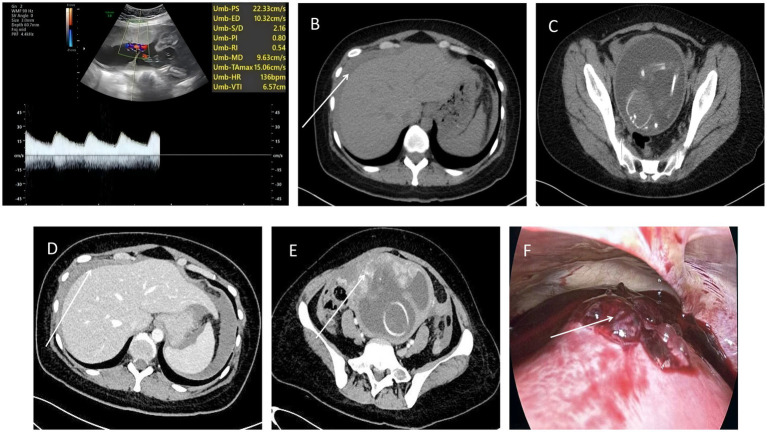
(A) Ultrasound indicates a single live fetus in intrauterine pregnancy. (B) A slightly high-density shadow can be seen around the liver and spleen, with a CT value of about 37–62 HU, which is considered as blood or fluid accumulation. (C) CT revealed the muscle layer and serosal layer are continuous. (D) There were many high-density fluid shadows in the perihepatic, perisplenic, bilateral paracolic sulci, mesenteric space, and pelvic cavity, suggesting massive hematoma or fluid accumulation in the abdominal and pelvic cavity. (E) The anterior wall of the uterus was slightly less clear. (F) Laparoscopy revealed a 4 cm × 1.5 cm rupture of the whole layer in the anterior wall of the uterus, 2 cm below the root of the round ligament (the abnormal region was indicated by the white arrow).
